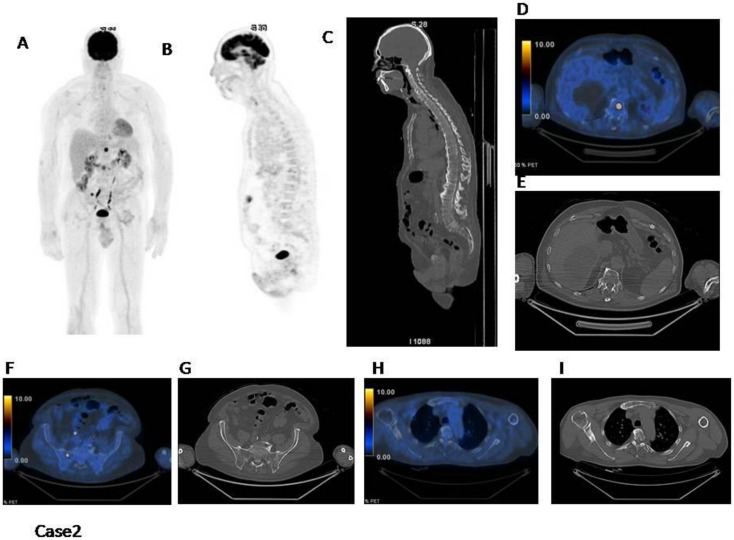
(A) MIP; (B) PET sagittal cut; (C) CT sagittal cut; (D–I) fused images and CT axial cut on different focal lesions. There are at least three focal hot spots with no underlying lytic lesions (D: vertebra, F: sacrum, H: right rib).
